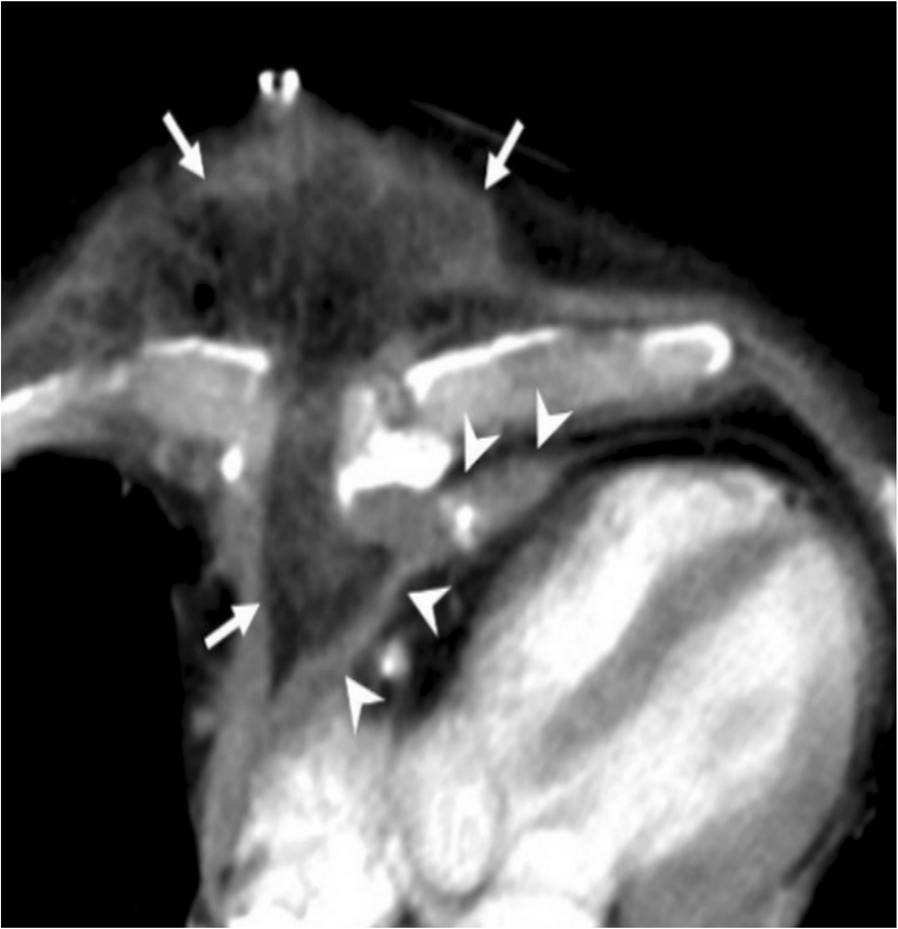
A 65-year-old man with a previous history of surgery for constrictive pericarditis developed chronic sternal osteomyelitis on the follow-up period. Axial contrast-enhanced CT image demonstrates a pedicled fat flap (arrows) placed to maintain hemostasis and infection control. Pericardial thickening (arrowheads) is also noted
